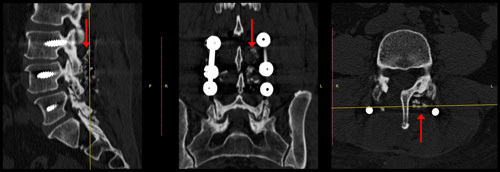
Spinal CT scans the day after surgery. On the left side (red arrows) is the autologous biological reinforcement bone powder for titanium-instrumented arthrodesis. CT, computed tomography.
